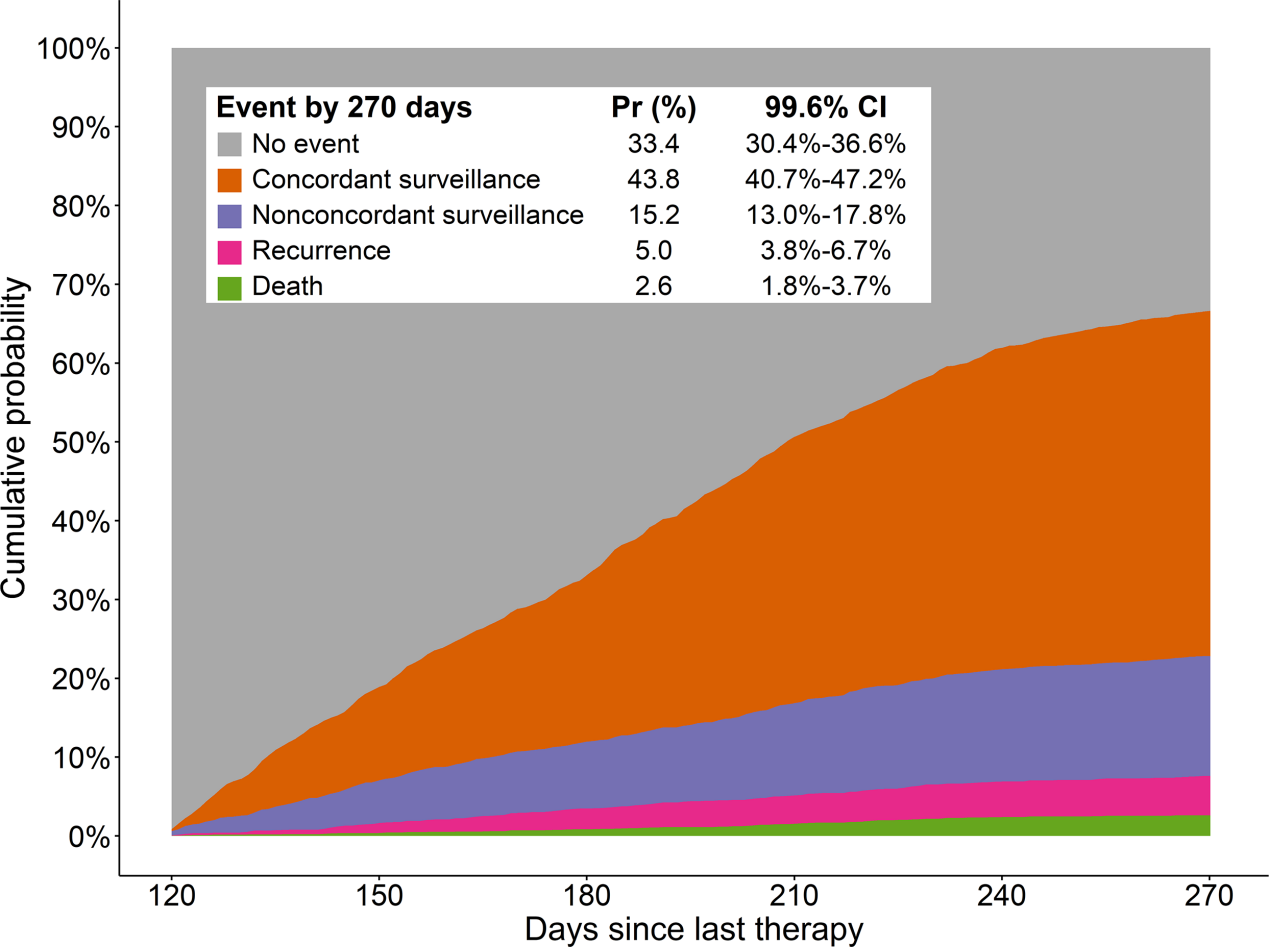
Cumulative probability estimated from an Aalen-Johansen competing events model for receipt of guideline-concordant imaging, defined as chest computed tomography imaging with a documented indication of surveillance, within 120 to 270 days after definitive therapy. The probability curves are stacked such that the sum at any time point is 100%. Patients were followed only until the first event, and cumulative probability is therefore the expected proportion of patients who had the event as their first event before a given time point. The overlaid table shows the cumulative probability (Pr) and 99.6% CI at 270 days after therapy.
